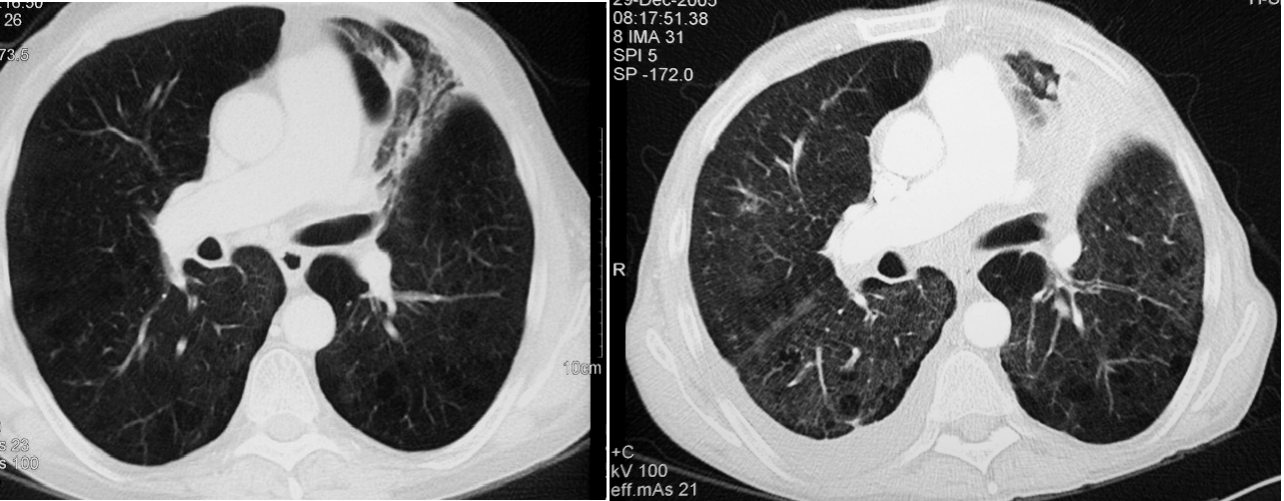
Chest CT scans before erlotinib therapy (left) and after two months of erlotinib therapy (right).
